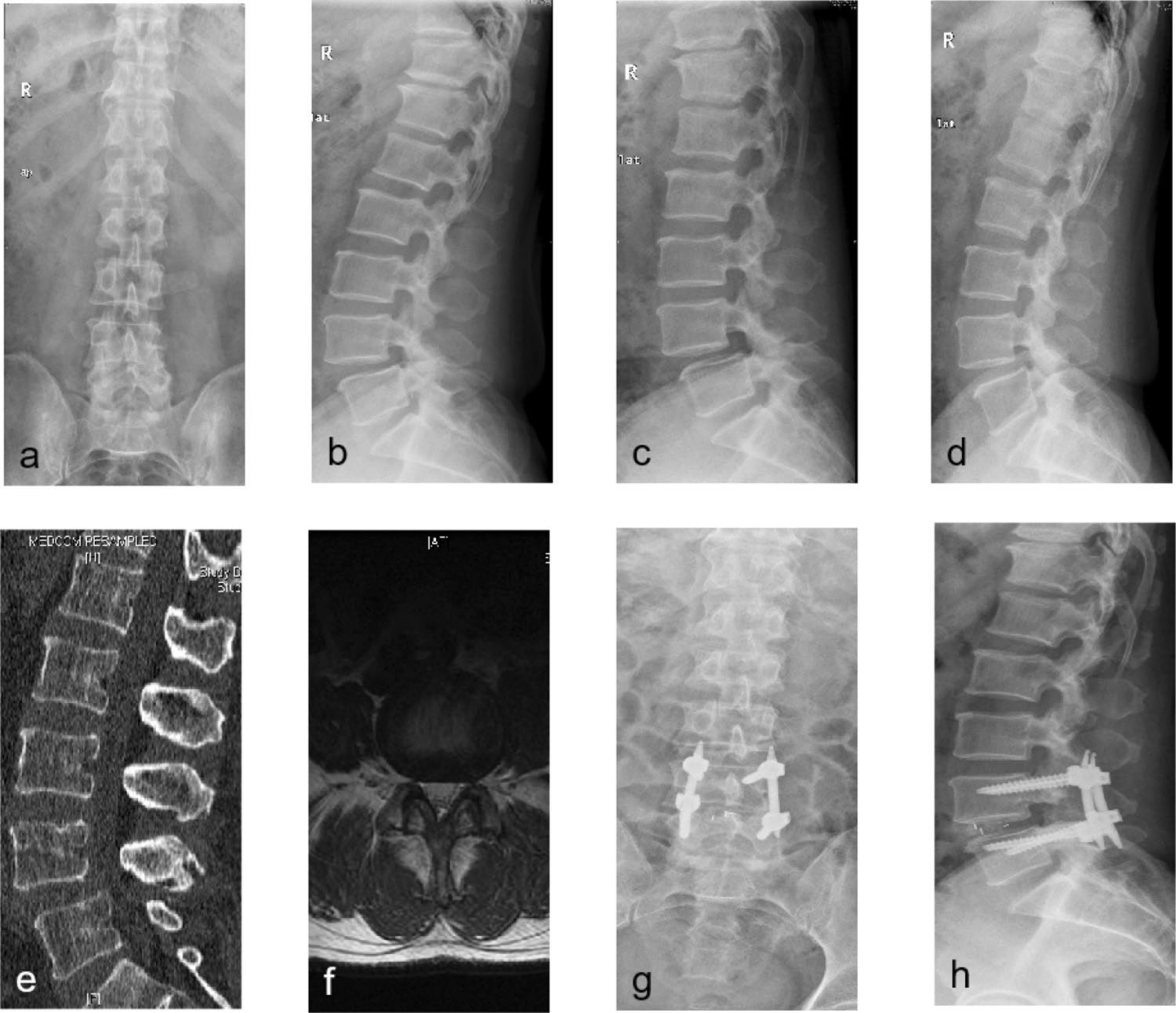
A 61 years old male patient who suffered from low-back pain for 16 months prior to admission underwent MIS-TLIF procedure. (a,b) Antero-posterior and lateral plain radiography before operation; (c,d) preoperative flexion and extension plain radiography indicated patient with LDS grade 1; (e,f) preoperative sagittal CT scan and axial T2-weighted MR image; (g,h) antero-posterior and lateral plain radiography after surgery.
